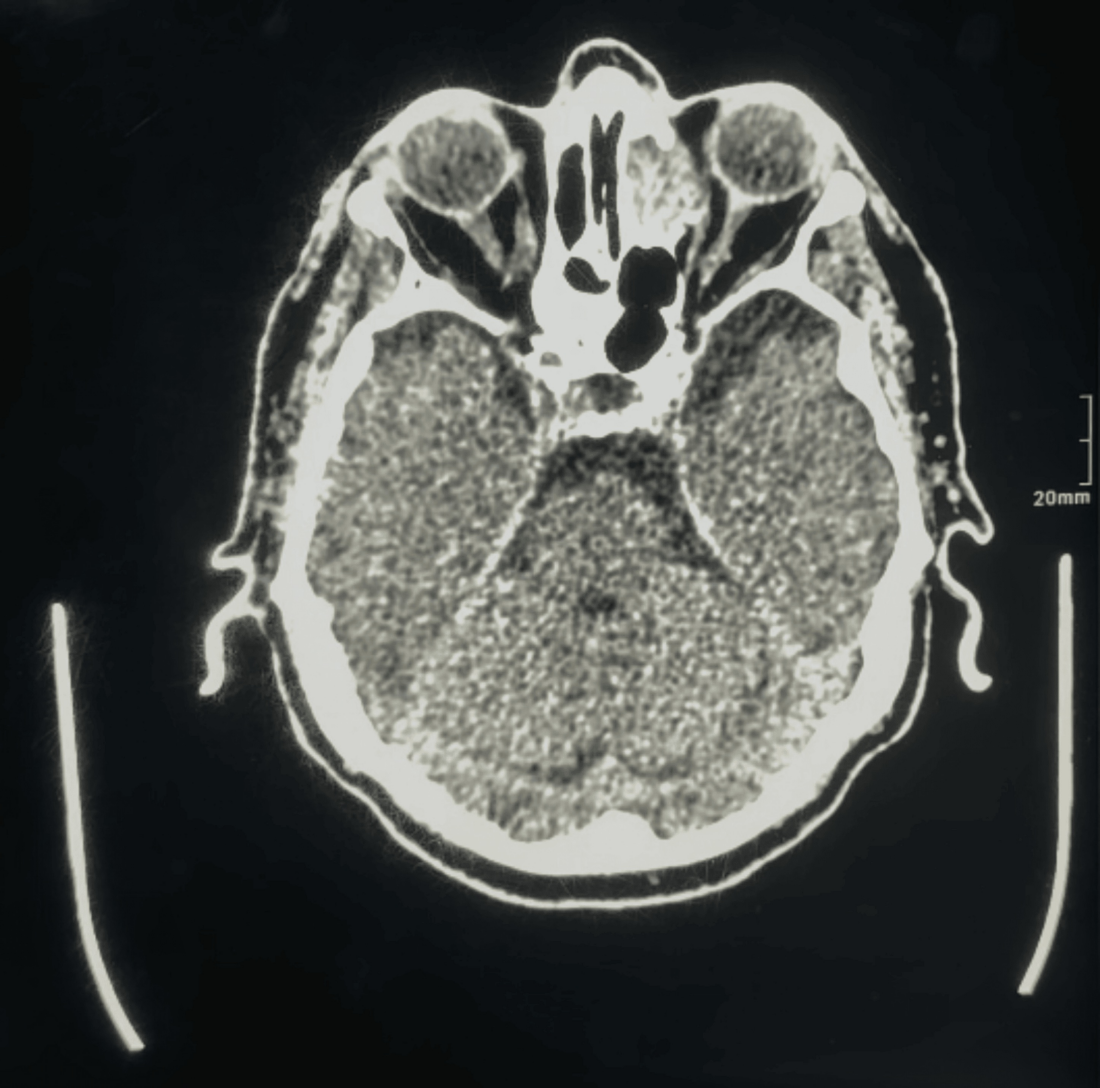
Preoperative sinus CT finding Opacification of the left anterior ethmoid air cell shows a bulge with underlying bone remodeling, lysis, and compression over the medial wall of the left orbit. It is abutting the medial rectus muscle. CT: computed tomography
